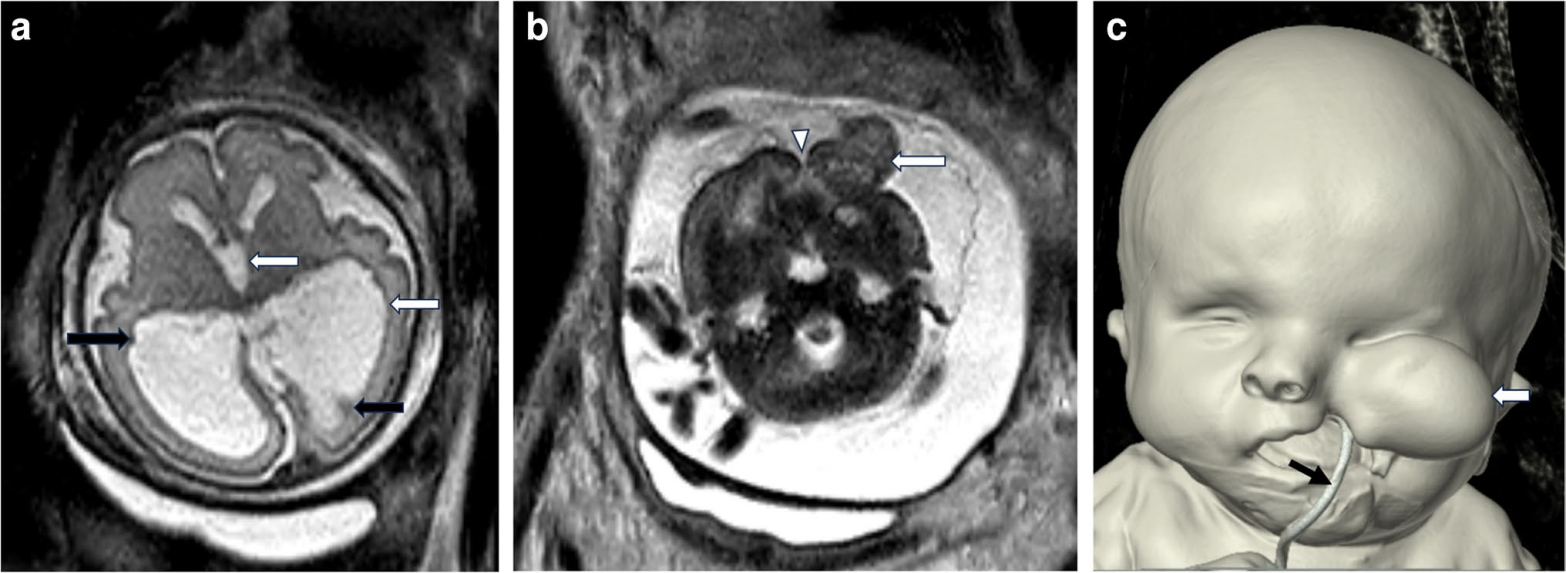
A 36-year-old gravid multipara woman was referred for a fetal magnetic resonance imaging (MRI) at 30-week gestational age after routine prenatal ultrasound revealed ventriculomegaly in one of the twins. a Axial T2-SSFSE image of the  fetal brain demonstrates ventriculomegaly involving bilateral lateral and third ventricles (white arrows), and multiple subependymal nodules (black arrows) consistent with periventricular gray matter heterotopia. b Axial T2-SSFSE image of the fetal face demonstrates left-sided cleft lip and cleft palate (arrowhead) and an extruding soft tissue lesion (arrow) arising from the left orbital/maxillary region with mixed T2 signal intensity. Postnatal MRI (not shown) confirmed these findings and additionally revealed a left closed lip schizencephaly. c Three-dimensional surface and volume-rendered reformatted computed tomography image demonstrates left paramedian cleft lip, palate and maxilla, and associated facial lesion (white arrow). Orogastric tube (black arrow), right microphthalmia, and nasal septal deviation are also noted
